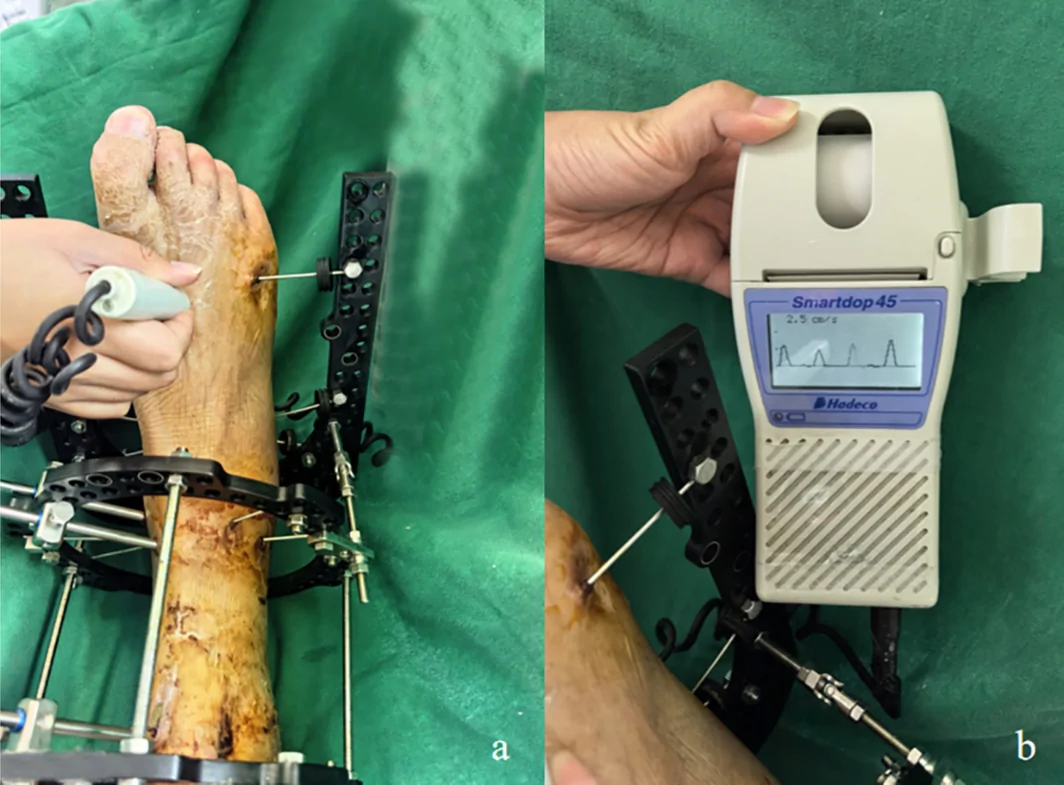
Intraoperative real‐time Doppler monitoring during acute shortening. (a) The portable Doppler probe is placed over the standard anatomical landmark of the dorsalis pedis artery on the ipsilateral foot. (b) The corresponding Doppler waveform shows a clear, uninterrupted arterial pulsatile signal at the maximal acute shortening point (up to 3 cm), confirming persistent distal perfusion.
